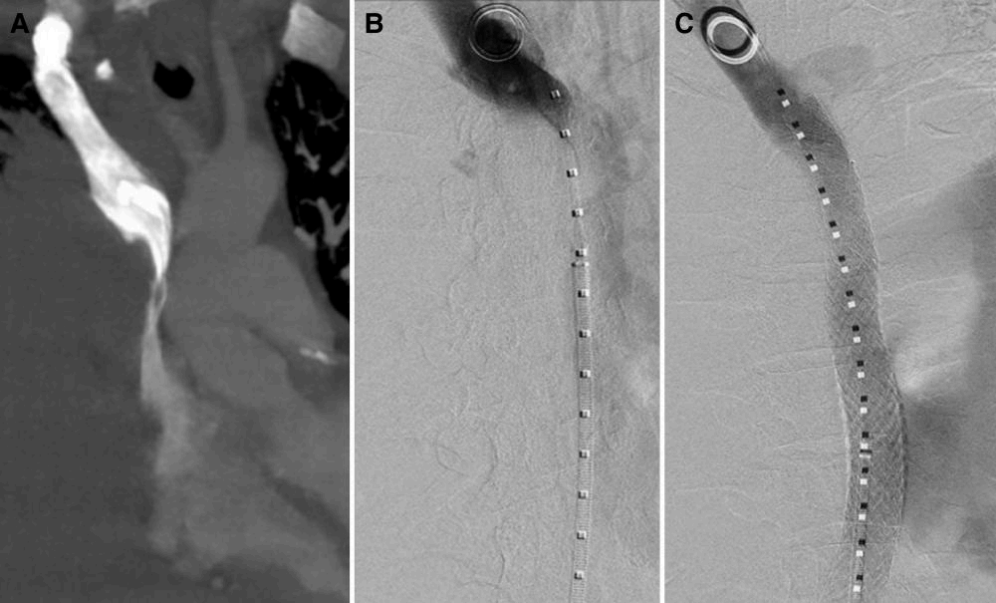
(A)* A female in her 70s with SVC syndrome due to large cell neuroendocrine carcinoma of the lung (patient 3). CT chest, maximum intensity projection reconstruction in coronal plane, shows diffuse stenosis of SVC extending to superior cavo-atrial junction. *(B)* Venogram shows a pigtail catheter and sheath from a right femoral approach with pigtail in the right brachiocephalic vein. There is contrast filling the right brachiocephalic vein without central flow due to diffuse stenosis of the SVC extending to the superior cavo-atrial junction. *(C)* Venogram post stent deployment shows relief of SVC stenosis with flow through the SVC-to-IVC bridging stent to the right atrium.
